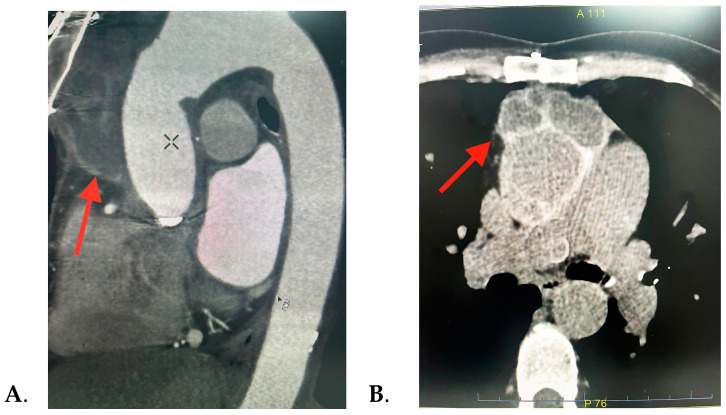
Thorax CT scan images showing the periaortic fluid accumulation (red arrow): (A) sagittal and (B) axial sections.
